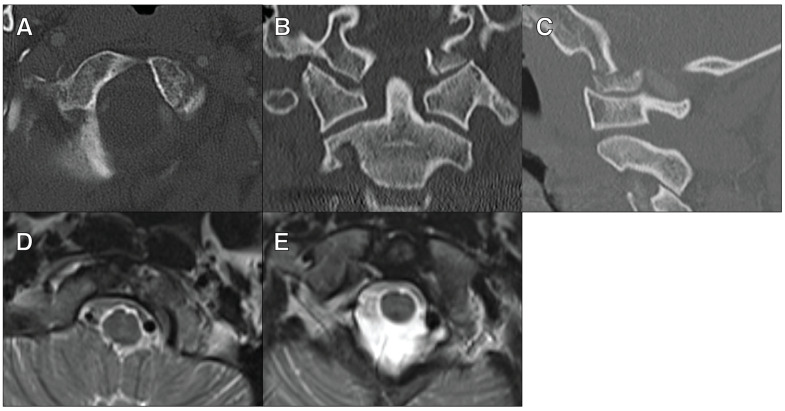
A 27-year-old male who presented after a fall from a scooter with a left type III OCF. (A) The axial view of a CT cervical scan showing the left type III OFC. (B) The coronal view of a CT cervical scan showing the left type III OFC and the coronal deformity. (C) The sagittal view of a CT cervical scan showing the left type III OFC. (D) The axial view of an MRI cervical T2 STIR sequence showing left alar ligament disruption. (E) The axial view of an MRI cervical T2 STIR sequence showing transverse cruciate ligament disruption.
